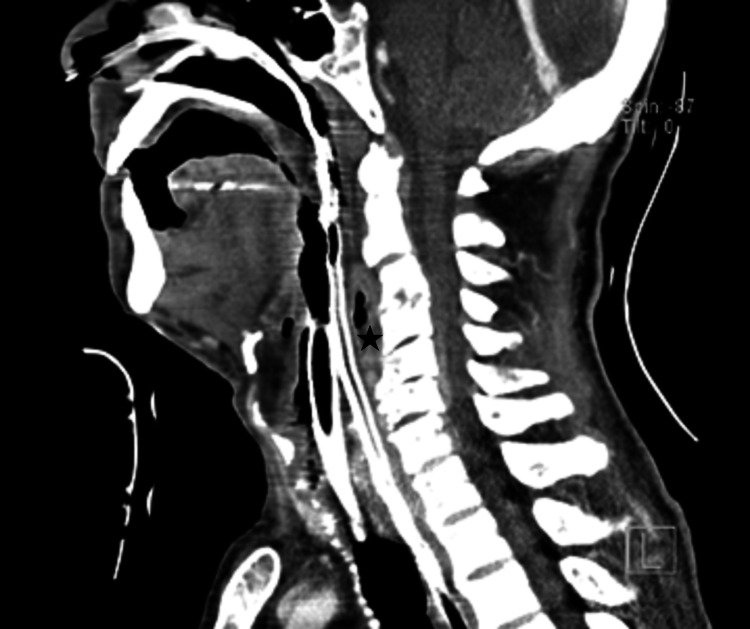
Postoperative cervical CT: the star shows the reduction of the retropharyngeal collection.
